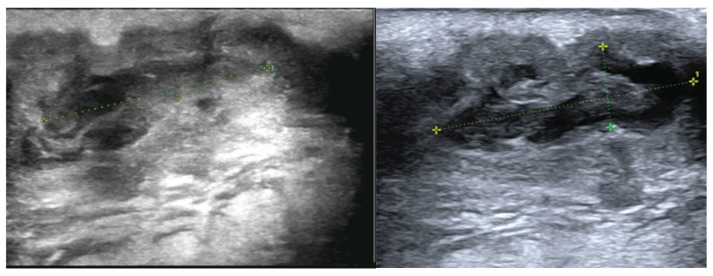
Ultrasonographic images of the cervical mass.
